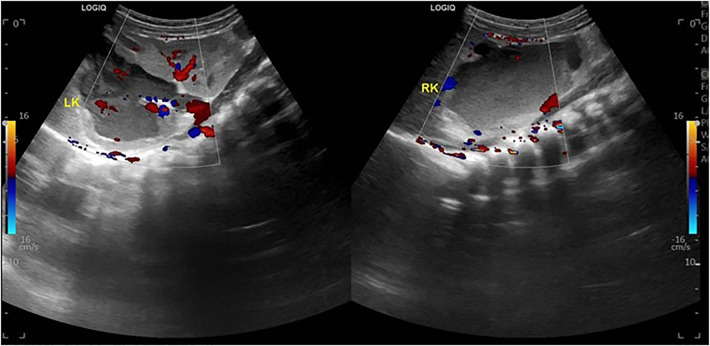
Preoperative ultrasound showed severe hydronephrosis and ureterectasis of bilateral upper moiety.
